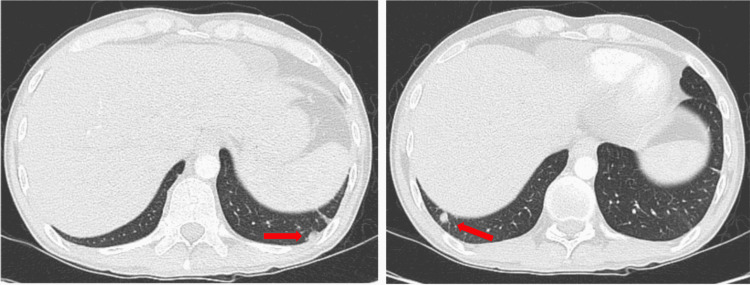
CTA of chest demonstrating multiple pulmonary nodules (red arrows). CTA: Computed tomography angiography.
